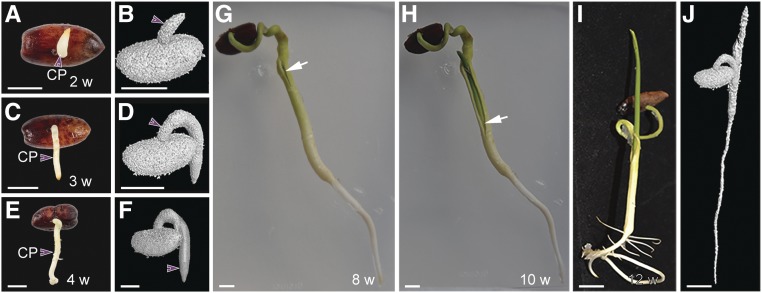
Germination Process in Date Palms. (A) to (J) Two- to 4 weeks after germination (see [A] to [F]), 8 weeks after germination (G), 10 weeks after germination (H), and 12 weeks after germination (see [I] and [J]). (A), (C), (E), and (G) to (I) are macrophotographs. (B), (D), (F), and (J) are images obtained from 3D x-ray computed tomography. The white arrows in (G) and (H) point to tissue apertures allowing leaves to emerge; purple arrowheads point to the cotyledonary petiole (CP). Images are representative of 40 seedlings. Bar in (A) to (J) = 1 cm. w, weeks after germination.
